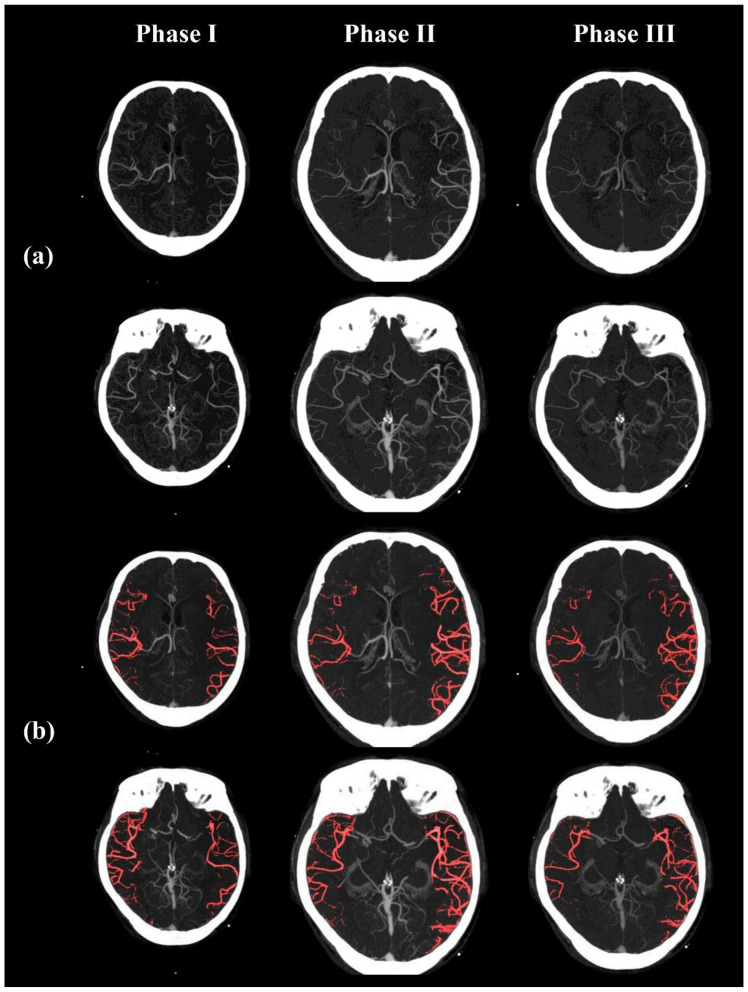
Example images of collateral vessel segmentation. It includes (a) original images of three phases of the ganglionic level and superganglionic level section and (b) an overlay of the original images and with segmented collateral vessels shown in red using the proposed method. The images are from a 73-year-old male patient with a left hemispheric large vessel occlusion. Visual collateral scoring yielded a score of 2 on single-phase CTA and 4 on multiphase CTA. Quantitative metrics were as follows: MaxFD = 1.075, delay indicator = 0.819, and mqCS = 0.880. The patient achieved complete reperfusion (TICI 3) and a favorable 90-day functional outcome (mRS = 0).
